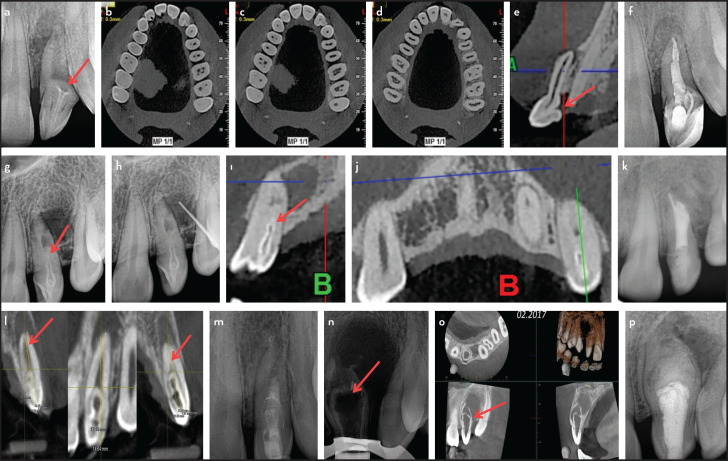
Different DI type II canal configurations. (a-f) Radiographs & CBCT images and post obturation radiograph depicting 3 canals in the coronal third (Type IIA). (g-k) Similarly type IIB with dens invading upto the middle third of the root canal. (l-p) Two type IIC canal configuration with dens extending to the apical thirds with 2 different treatment approach; revascularization (l, m) and Biodentine apical plug (n-p)
